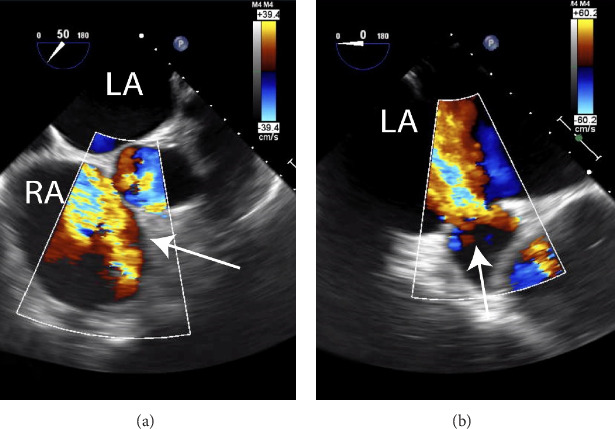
(A) Transesophageal echocardiogram (TEE) showing the mid-esophageal modified right ventricular inflow view. There is severe tricuspid regurgitation (marked with arrow). (B) TEE showing a lower esophageal view of the inferior sinus venosus atrial septal defect (ASD). This image shows a high-velocity jet with right-to-left shunting. This is the result of the traumatic severe TR and the jet is directed at the sinus venosus ASD causing the right-to-left shunting (measuring 75 and <10 mm^2^).
